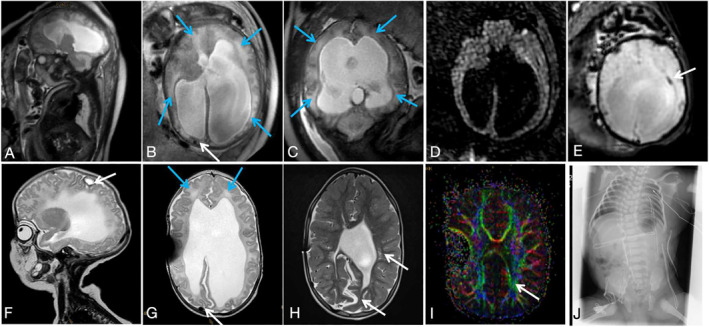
Phenotype of Chiari II malformation with brain edema and intracranial hemorrhage. (A–E) Prenatal MRI performed at 37 + 2 weeks shows the herniating cerebellum protruding posteriorly to the occiput bone (A), global brain parenchyma edema (blue arrows in B and C), and shifting and compressed superior sagittal sinus (B, white arrow). No hyperintensity region is shown on the DWI sequence within the parenchyma (D), indicating vasogenic edema, and the EPI‐T2* image shows ependymal hemorrhage at the left posterior horn (arrows in E). (F, G) Follow‐up postnatal MRI performed at 7 weeks of age (after postnatal MMC repair surgery and EVD shunt) shows cystic lesions with blood breakdown products (F, arrow), residual edema (blue arrows), and the shifting superior sinus (G, arrow). (H) Postnatal MRI performed at 5 years of age, shows a typical absence of the septum pellucidum and hemispheric interdigitations, periventricular white matter dysplasia, and volume loss (arrow). (I) DTI showed dysplasia of the white matter (arrow). (J) An X‐ray performed at 2 months of age showed hemivertebrae formation, bifurcated ribs, and aplasia of the 12th pair of ribs, as well as scoliosis.
